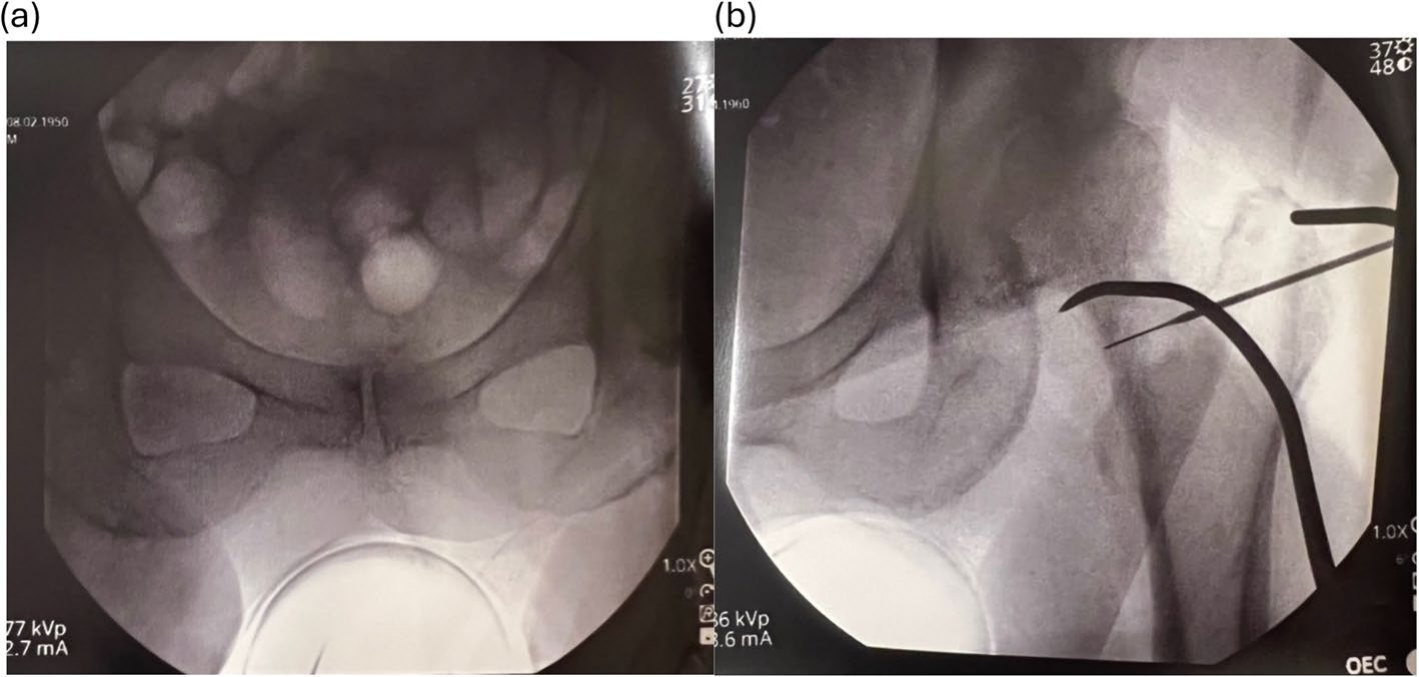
Intraoperative image intensifier radiographs of the leveled pelvis (a) and AP of hip (b) are obtained for surgical navigation with the ROSA hip system
